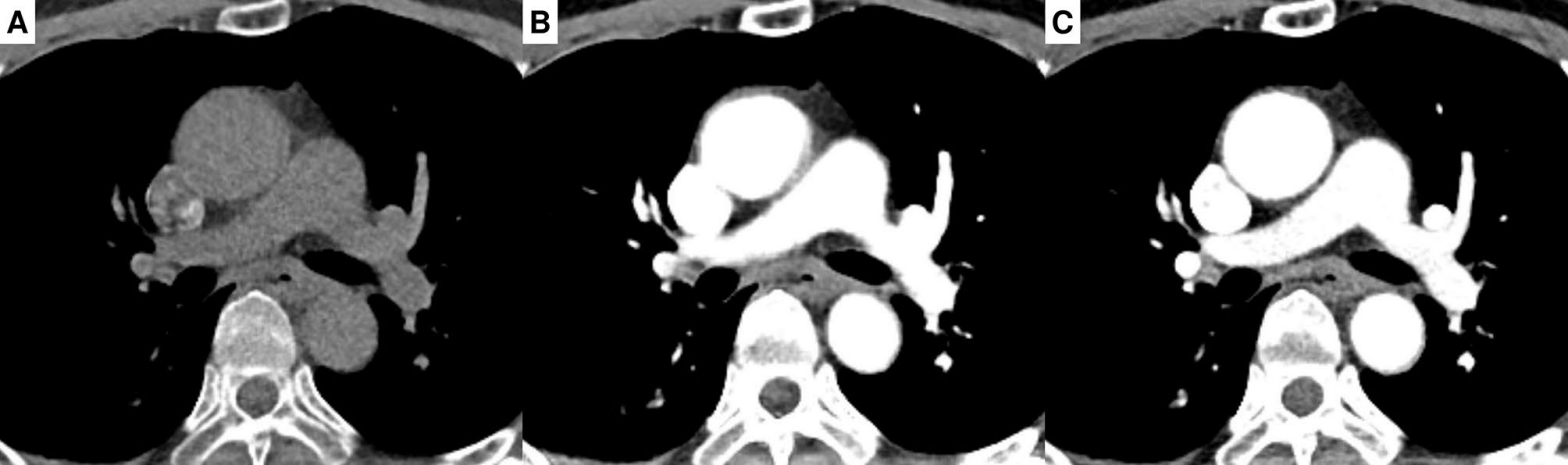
Images of a 63-year-old woman with right pneumothorax from the tuning set are presented. Non-contrast CT (A), synthetic contrast-enhanced CT (B), and contrast-enhanced CT (C).
